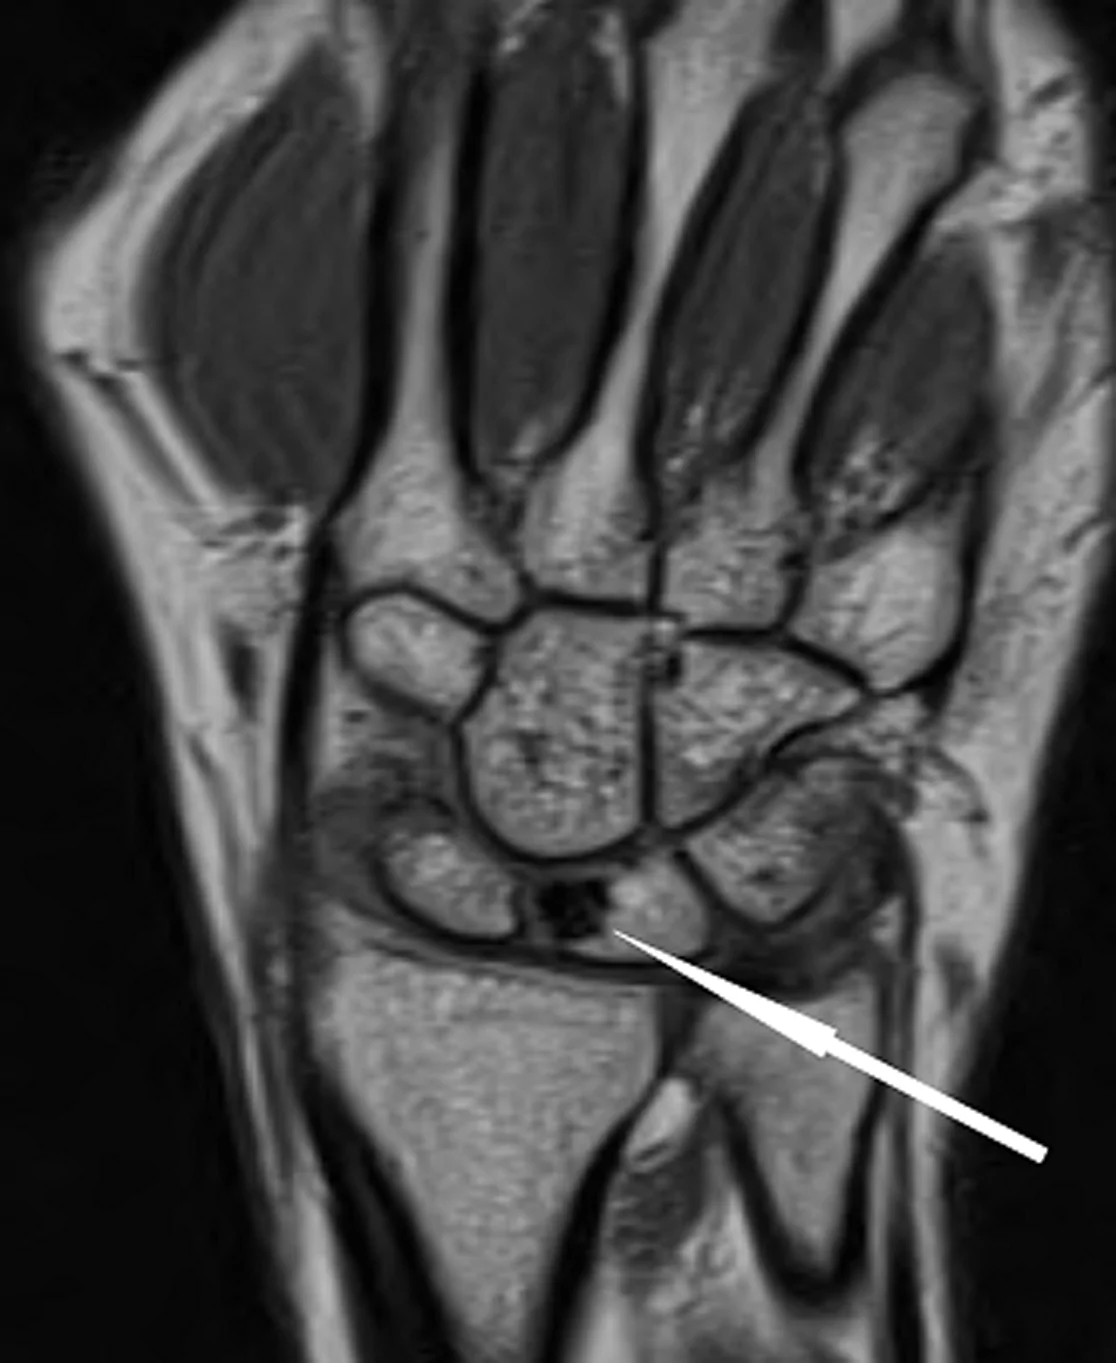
coronal T1-weighted MRI of the wrist demonstrates a focal hypointense area in the lunate (arrow), consistent with chronic avascular necrosis
